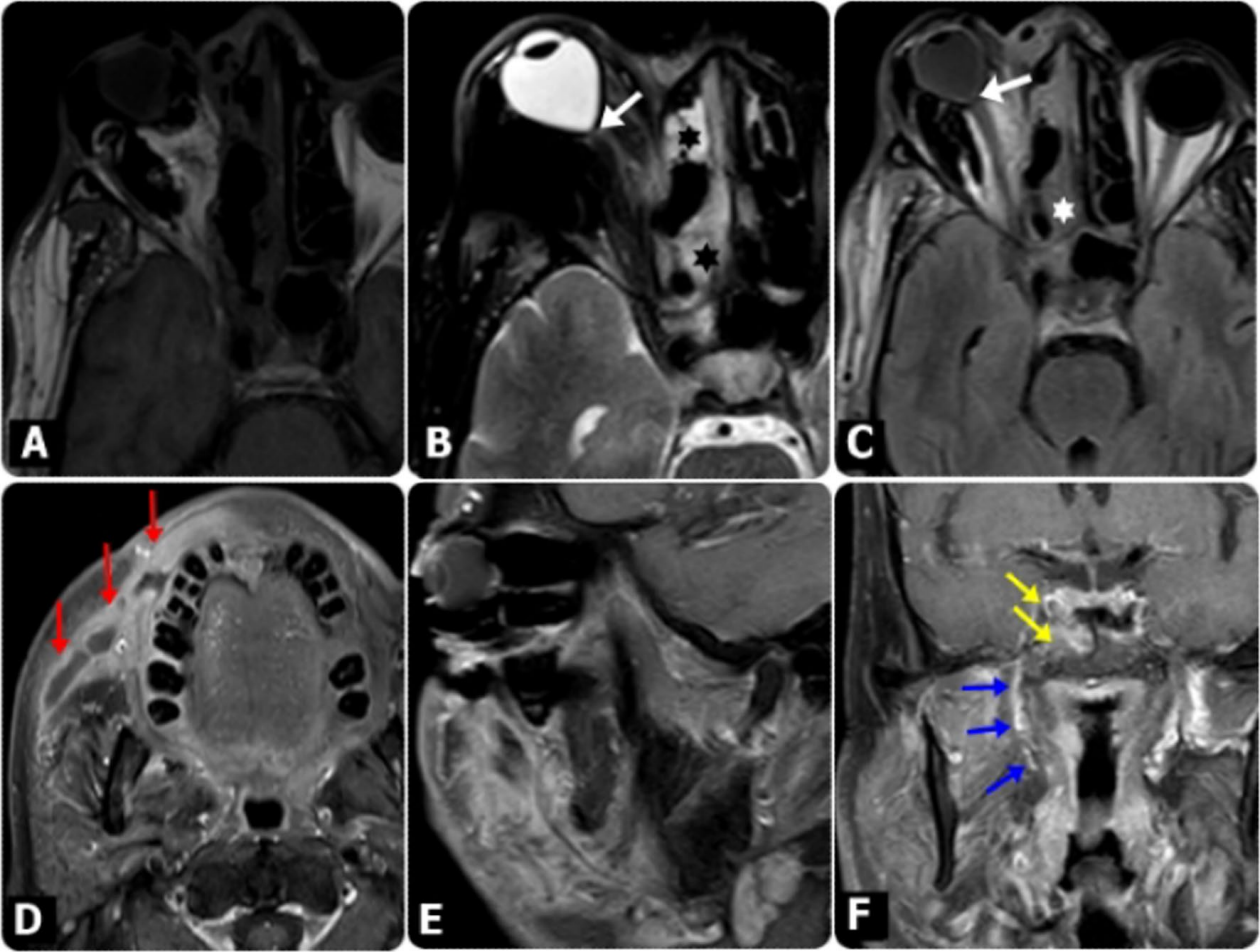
MRI findings in diabetic male patient, 49 years old, presented mainly with Rt. eye proptosis and Rt. premaxillary and periorbital edema, following COVID-19. A Axial T1W, B Axial T2-SPIR and C Axial FLAIR images showed ethmoidal sinusitis (Asterisks), more evident at the Rt. side, with extension of the inflammatory process to the Rt. orbit resulting in proptosis of Rt. eye globe, with posterior globe tenting (White arrows), as well as Rt. periorbital and eyelids edema. D Axial and E Sagittal post-contrast fat-saturated T1WI showed extension of the inflammatory process to the Rt. premaxillary region resulting in soft tissue edema and to the Rt. maxillary and zygomatic bones, showing heterogeneous post-contrast enhancement in keeping with bony invasion (red arrows). The edema and inflammatory process seen extending to the Rt. retro-maxillary region showing mild soft tissue contrast enhancement. F Coronal post-contrast fat-saturated T1WI showing mild enlarged right cavernous sinus with patchy heterogeneous enhancement (yellow arrows), with inflammatory spread to the Rt. infra-temporal fossa region (blue arrows)
